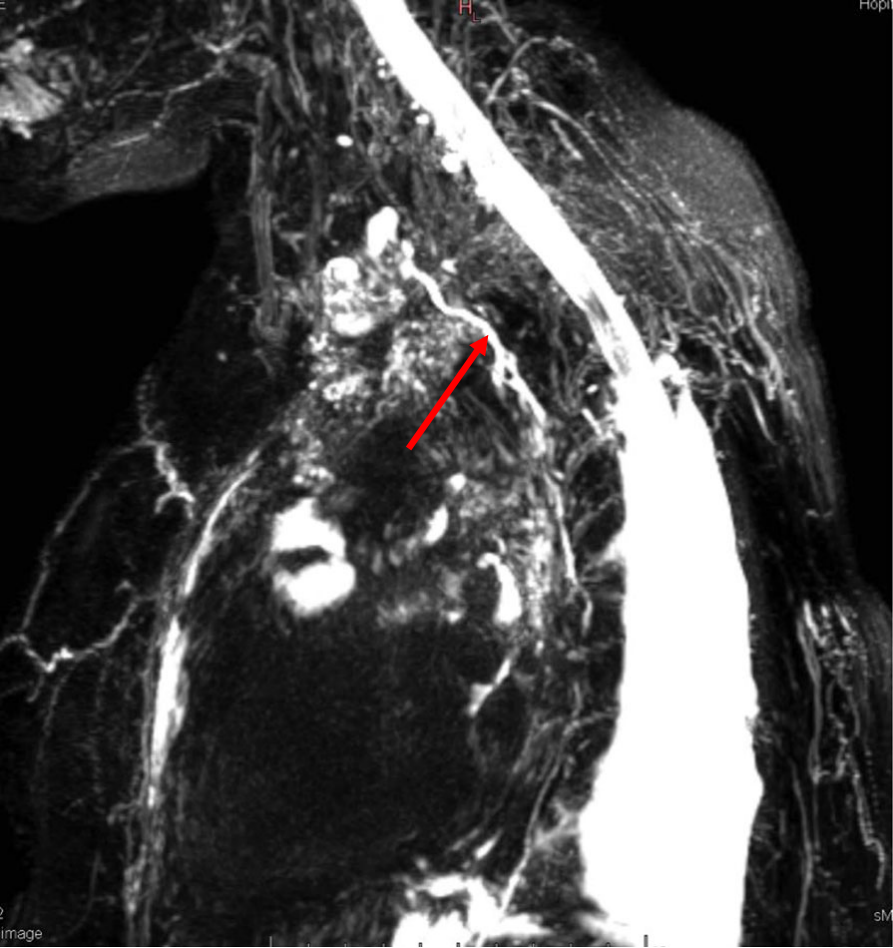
Axial MRI T2 showing the thoracic duct (*red arrow*)
